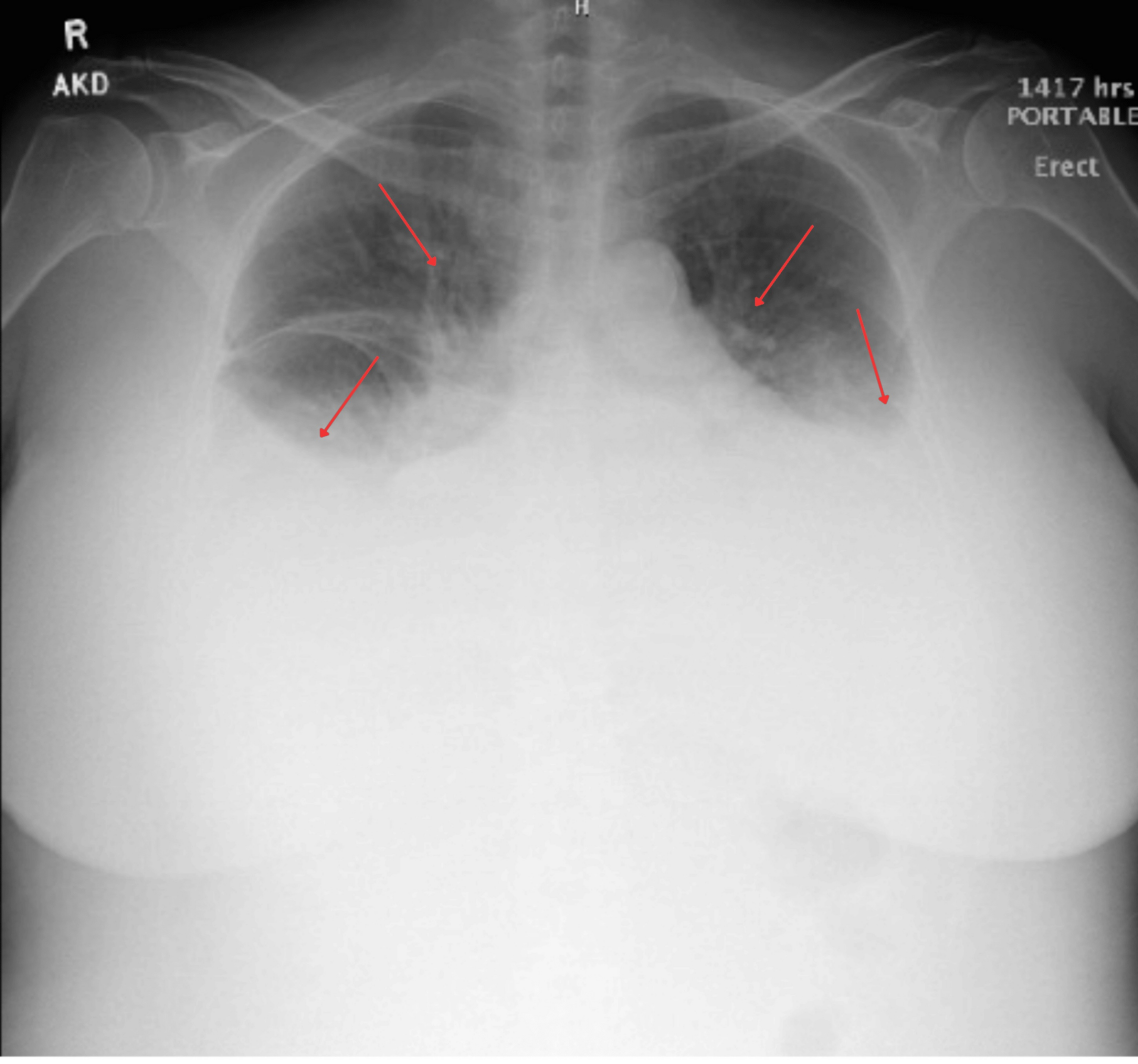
Chest X-ray demonstrating moderate pleural effusions with pulmonary congestion An upright, portable anteroposterior chest radiograph shows bilateral blunting of the costophrenic angles and layering fluid, indicated by red arrows, consistent with moderate pleural effusions. There is also an increased perihilar and interstitial opacity, suggesting pulmonary vascular congestion.
